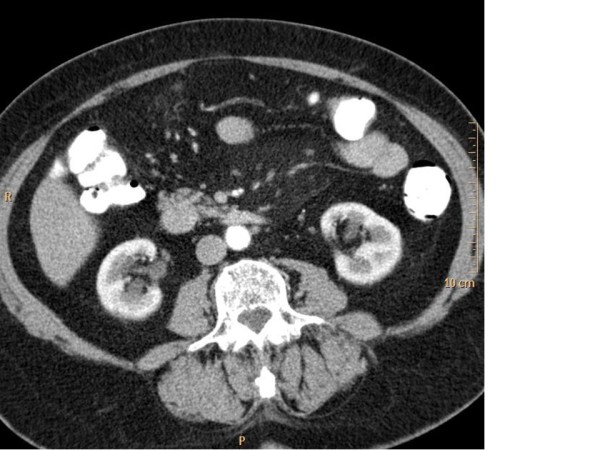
CT findings of mesenteric panniculitis. Enhanced abdominal CT at the mid-abdomen shows a hypertrophied fatty tissue in the mesenteric root with slightly increased attenuation delimited by a linear hyperdense rim (pseudo capsule). Some small nodules along the mesenteric vessels corresponding to lymph nodes but no mesenteric nodules of solid non fatty tissue.
